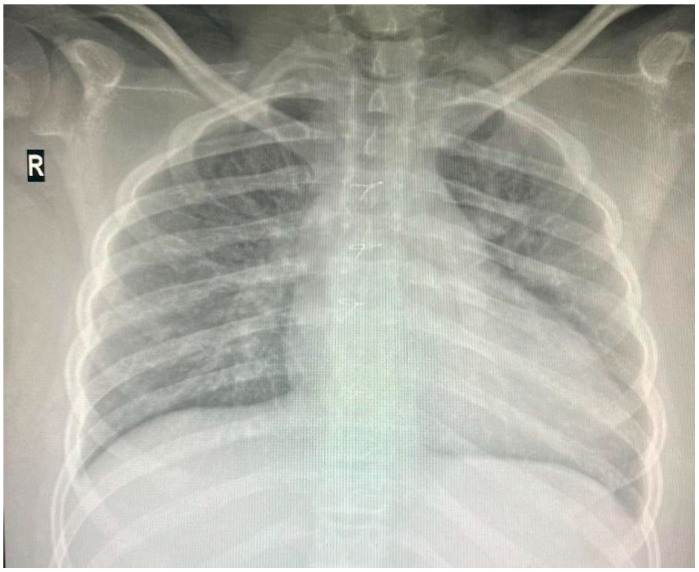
Thoracic X-ray: cardiomegaly, boot-shaped heart.
